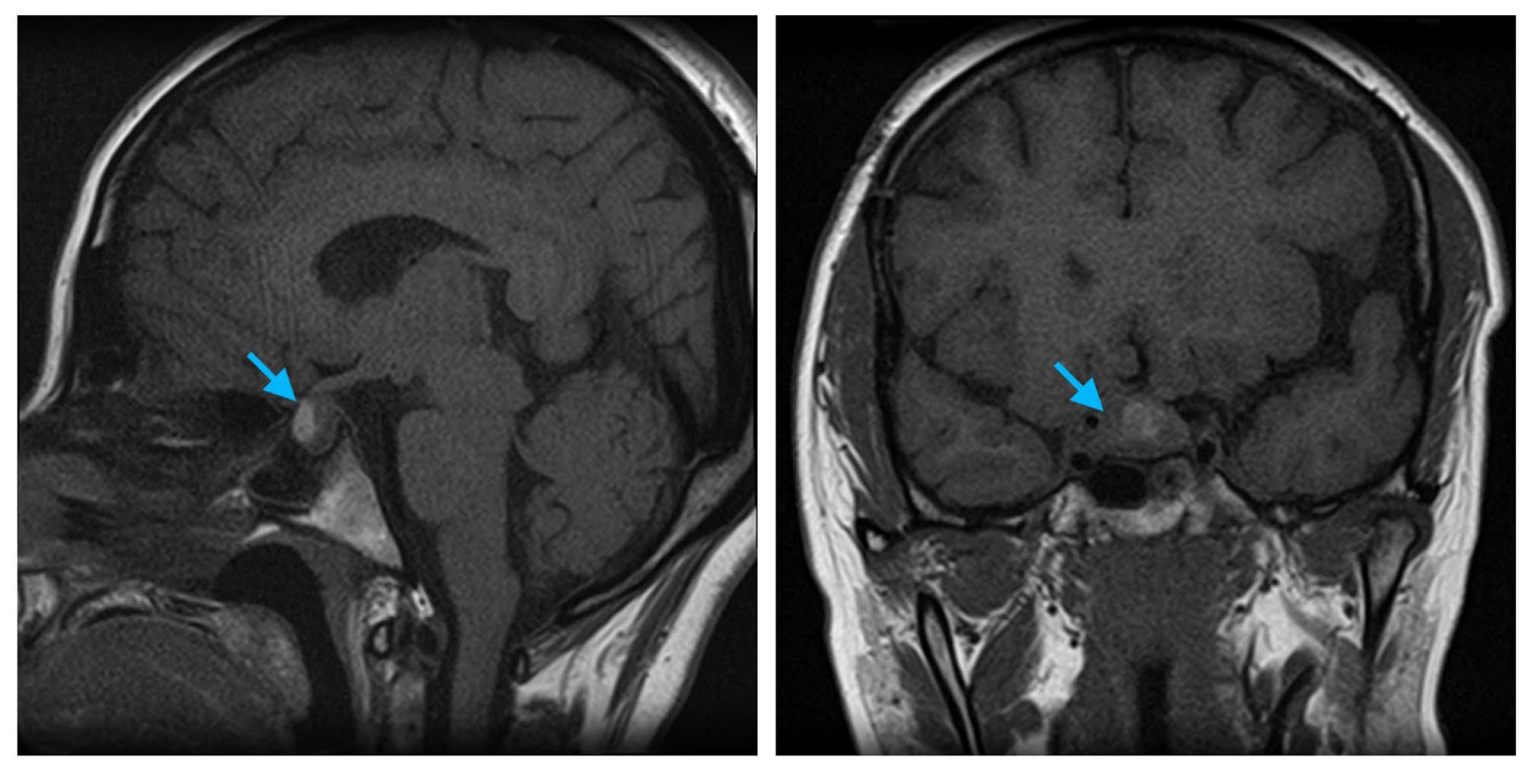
Blue arrow points to the inflamed pituitary gland, 6-month post-op MRI. Compared to pre-op MRI, post-op MRI shows a redemonstration of a sellar mass, similar in size, with mild mass effect on the optic chiasm. (A) Sagittal view of head MRI T1 without gadolinium contrast. (B) Coronal view of head MRI T1 without gadolinium contrast
